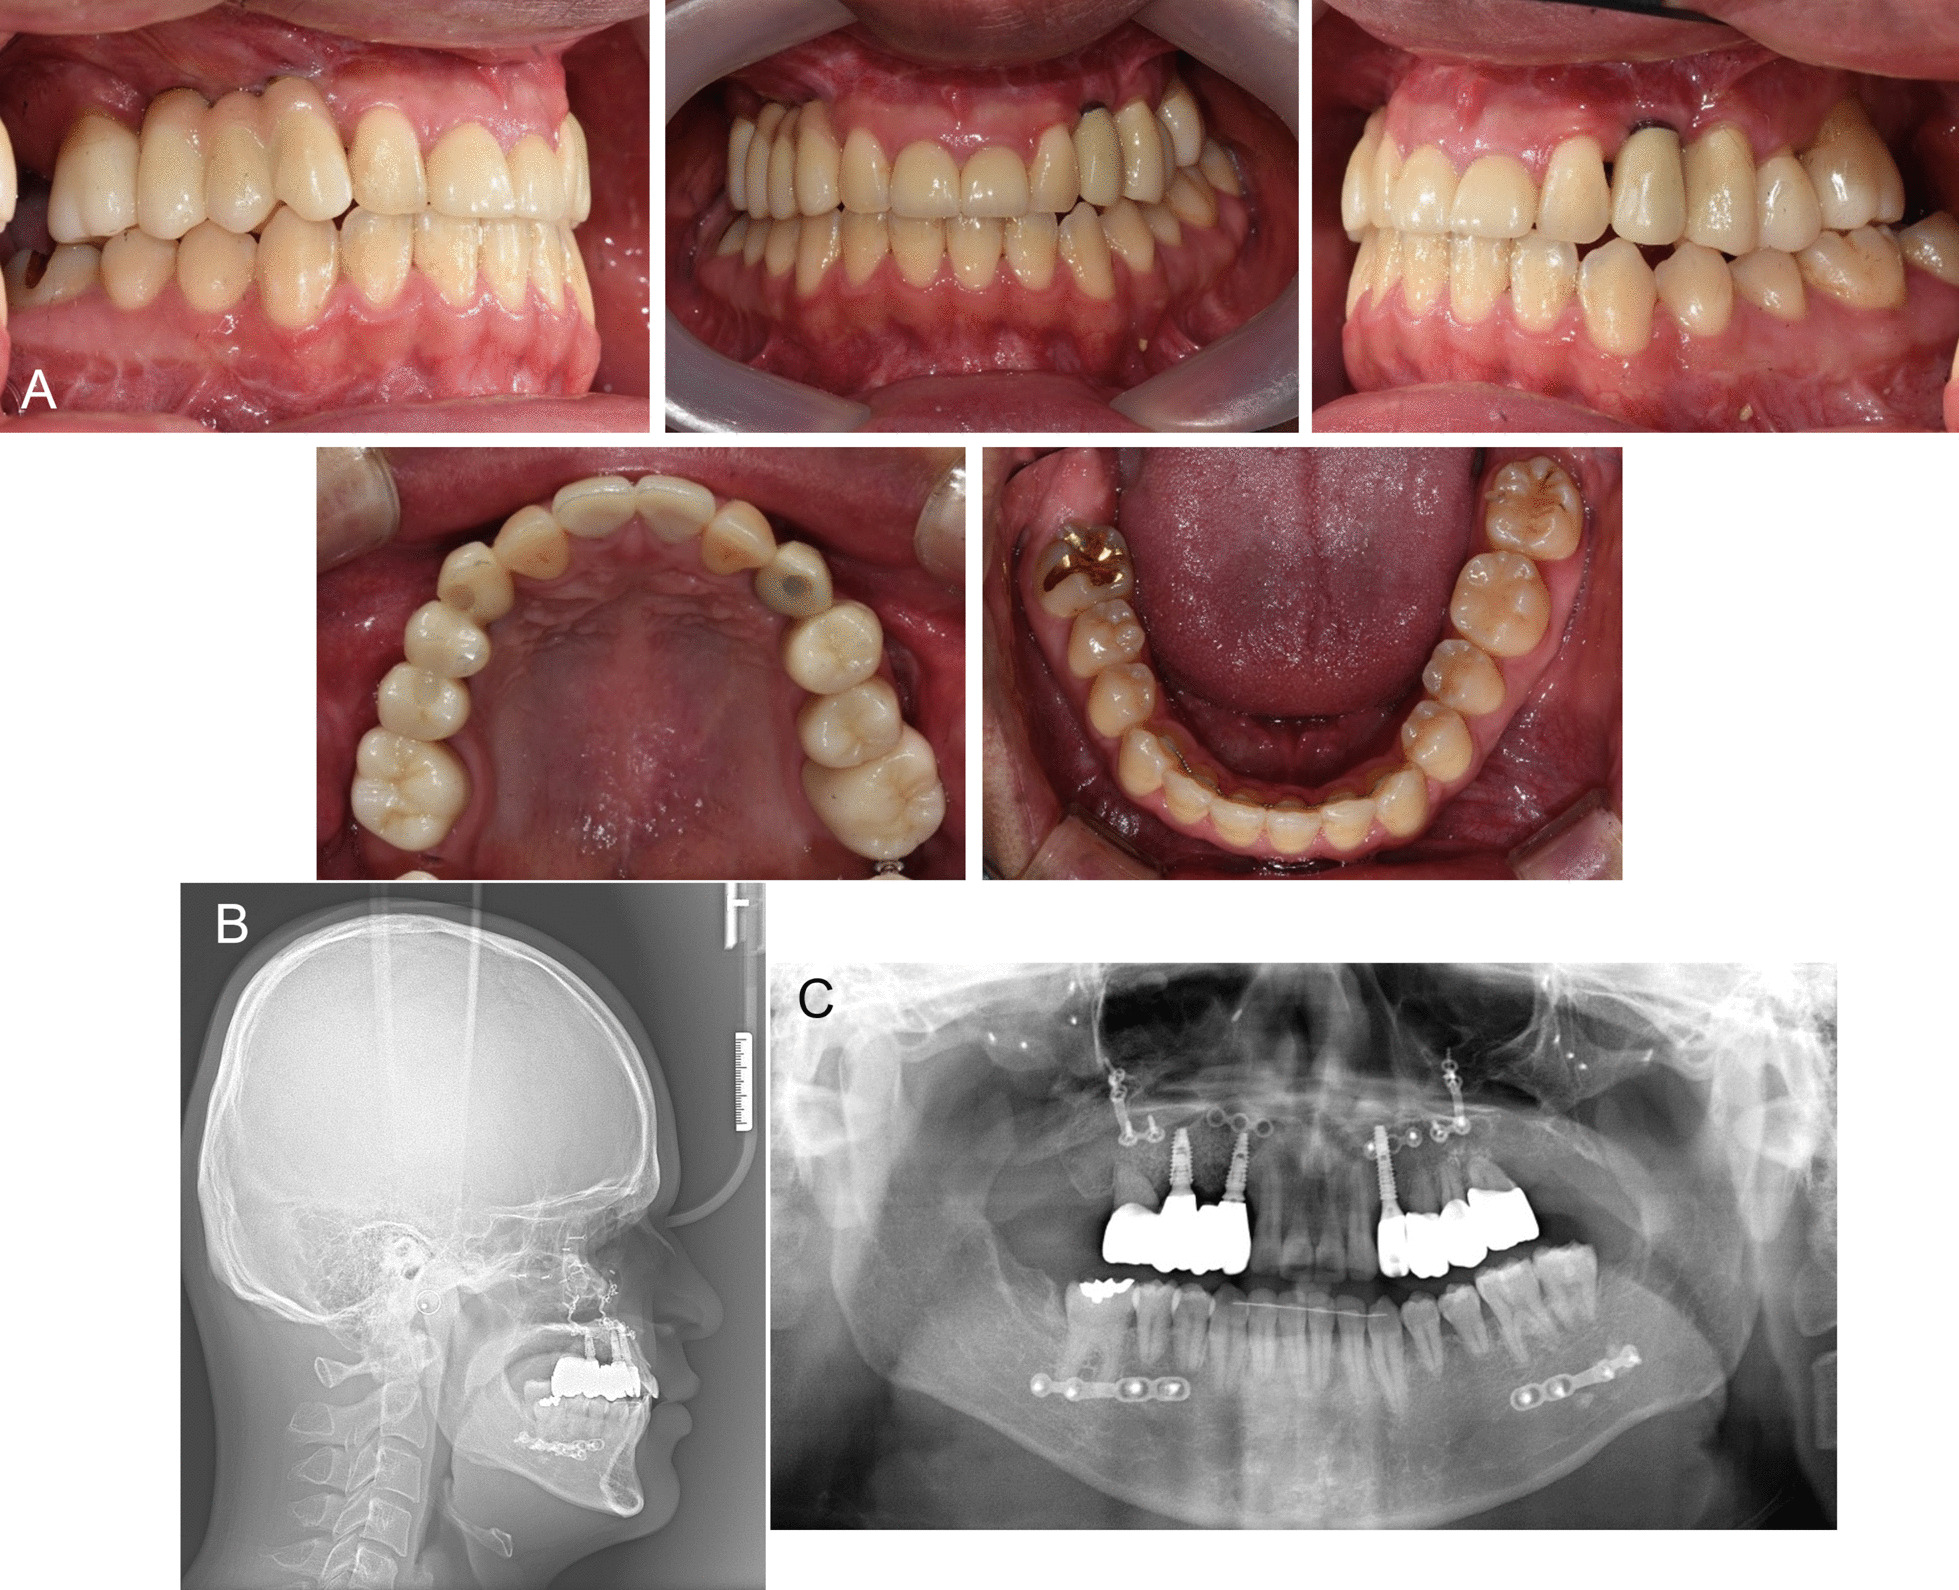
Two-year follow-up records of the patient. A. Intraoral photograph; B. Lateral cephalogram; C. Panoramic radiograph.
